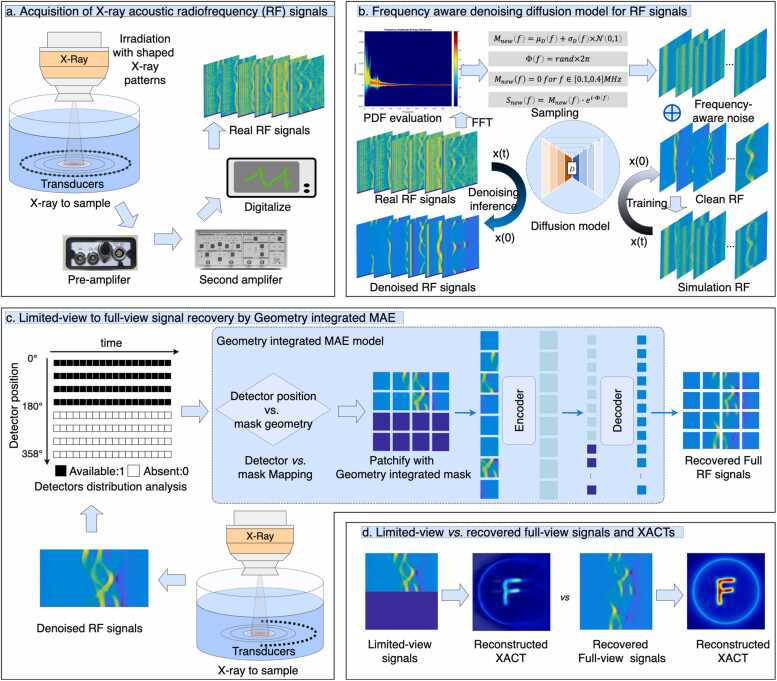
Overview of the proposed frequency-aware denoising and geometry-integrated masked autoencoders for limited-view signal recovery in X-ray Acoustic Tomography (XACT).
